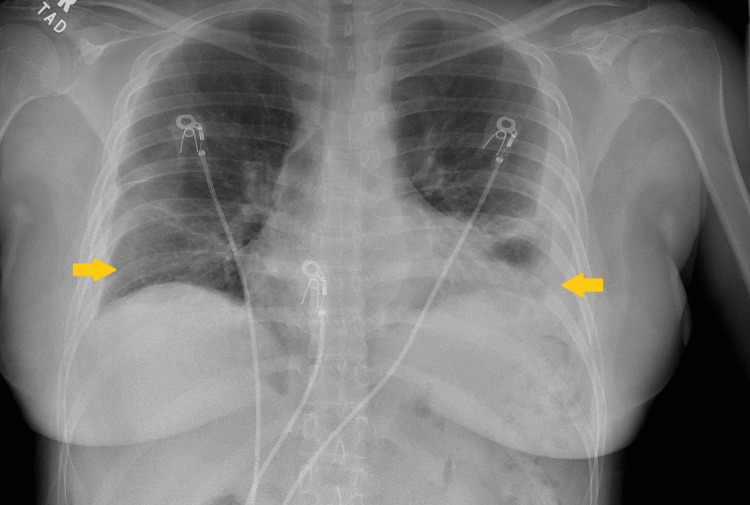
A chest X-ray from the initial hospitalization showed a small right-sided pleural effusion and a moderate left-sided pleural effusion.
